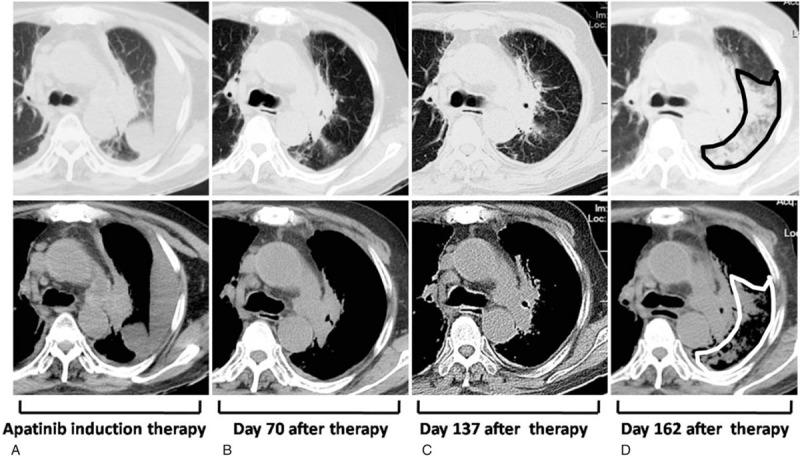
Computed tomograms of the patient after apatinib therapy. (A) Prominent pleural effusion in the left chest of the patient. (B) and (C) the effusion in the left chest almost completely disappeared. (D) Extensive inflammatory infiltration (see the marked area) in the left chest.
